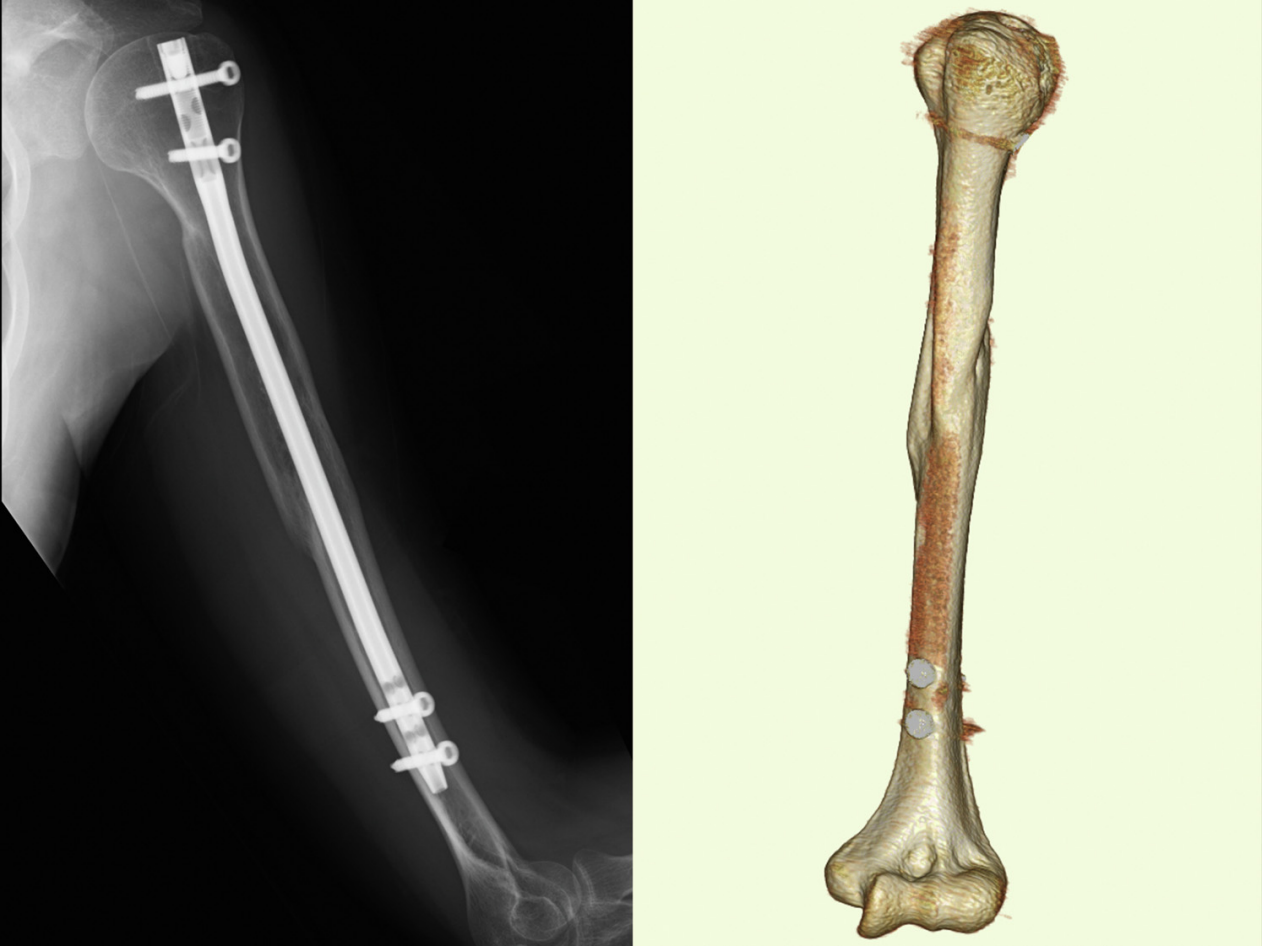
Plain radiographs and CT scans 8 months after surgery showing rotational deformity with 118° of humeral retroversion between the humoral head and elbow axis. *CT*, computed tomography.
